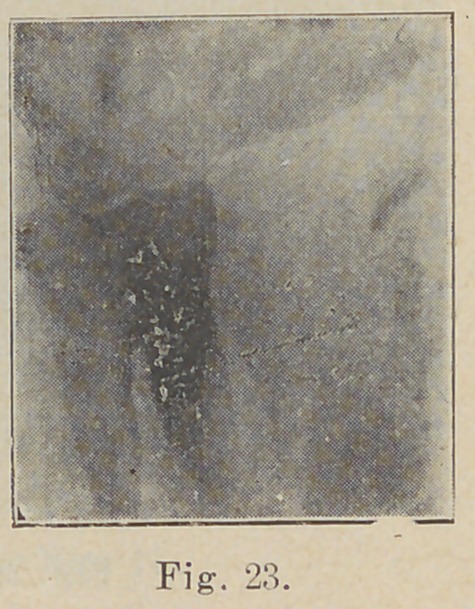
# The Roentgen Rays with Associated Phenomena and Their Applications in Dentistry
